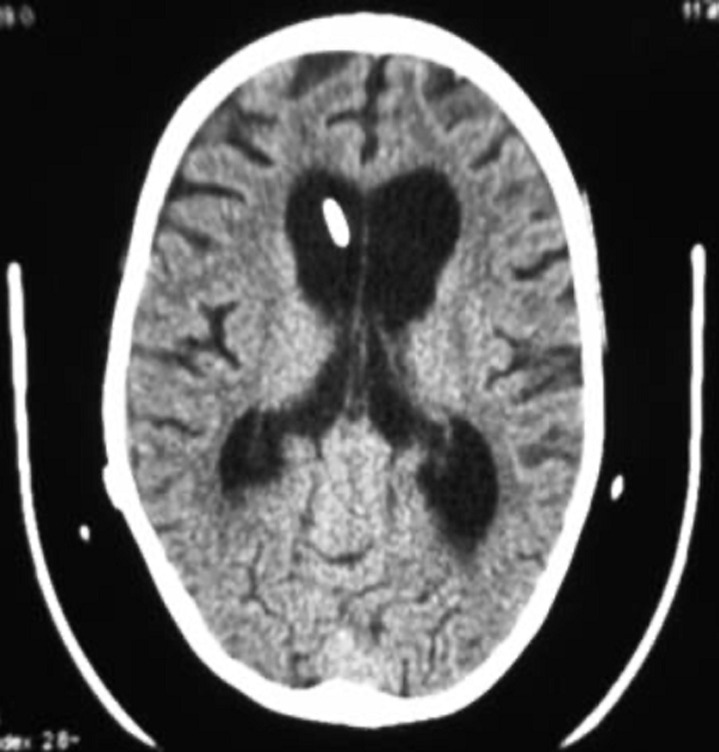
the cerebral CT scan also shows the right frontal ventriculoperitoneal shunt valve in place, with normal-sized ventricles
